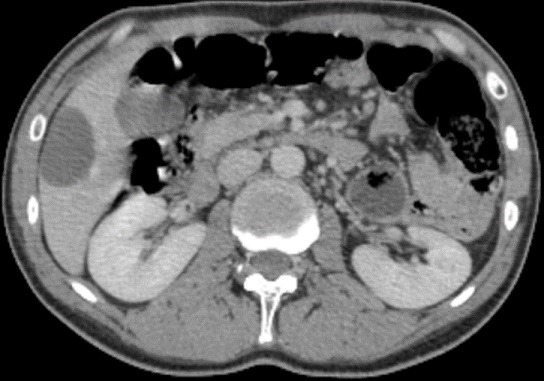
Abdominal CT scan showing a partial response of the liver metastasis to treatment
